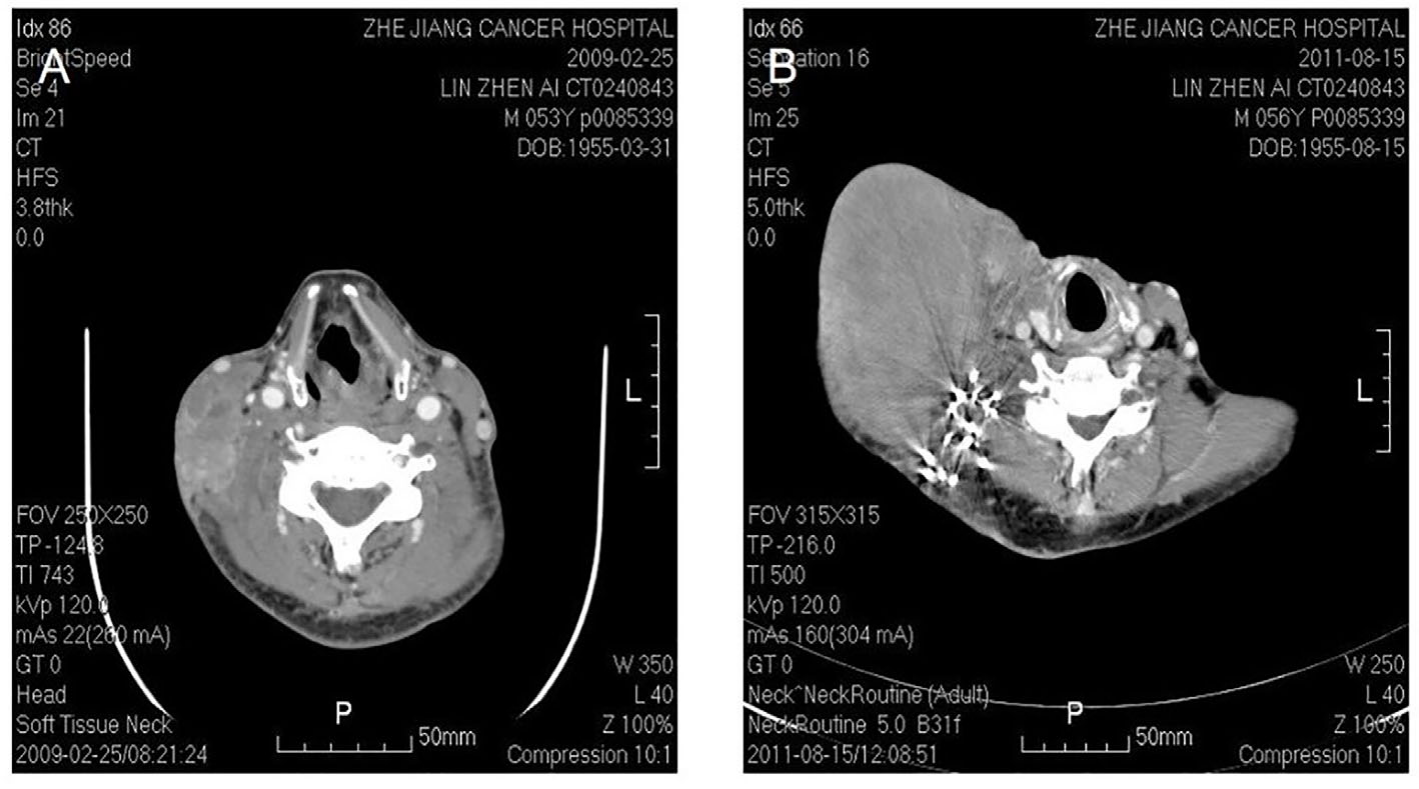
Malignant fibrous histocytoma in right neck. CT shows soft tissue image nearby sternocleidomastoideus in the middle to lower portion of right neck, with illegible margin and patchy enhancement after contrast. The lesion violates circumambient skin, subcutaneous tissue and sternocleidomastoideus (A). The picture on the right shows that recurrent lesion with superficial elcosis is surrounding the right cervical vascular, treated by high density particle (B).
